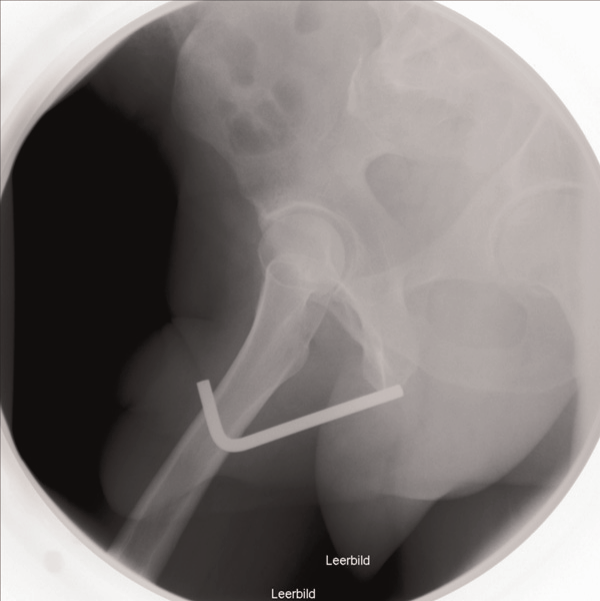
X-ray of the pelvis showing the Allen key completely in the anterior urethra.
